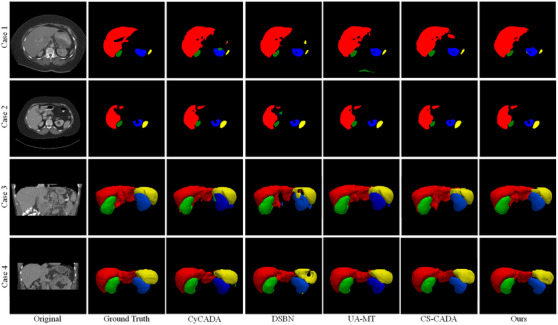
Comparative visualization of computed tomography (CT) segmentation in domain adaptation from magnetic resonance imaging (MRI) to CT. The liver, the right kidney, the left kidney, and the spleen correspond to red, green, blue, and yellow, respectively.
